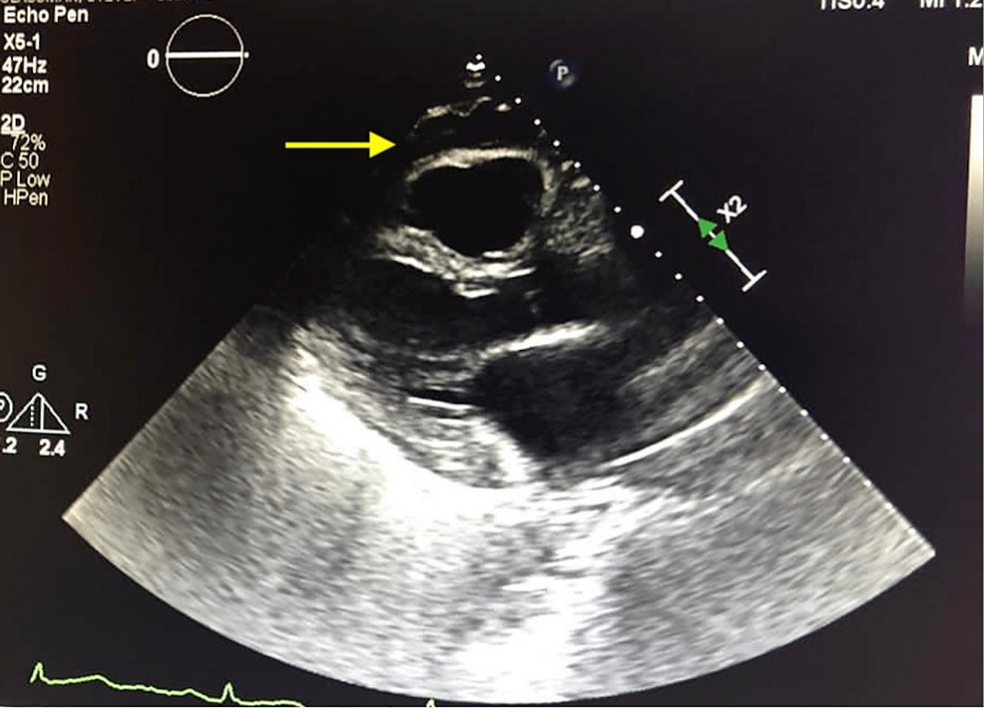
2D transthoracic echocardiogram post pericardiocentesis with removal of 750 cc of serosanguinous fluid
